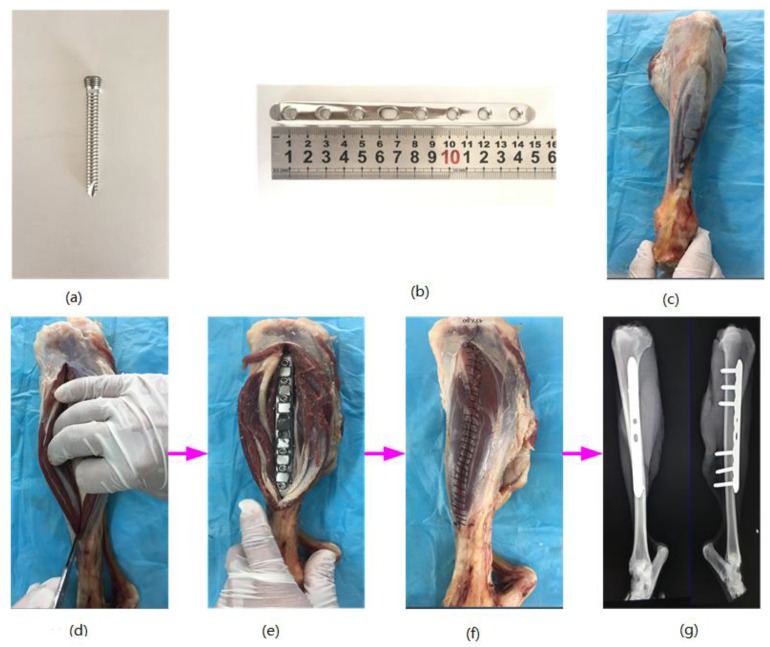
The installation process of the bone plate. (a) the bone plate screw; (b) the bone plate; (c) preparation; (d) cutting open; (e) implantation; (f) suture; (g) the X-ray image.
